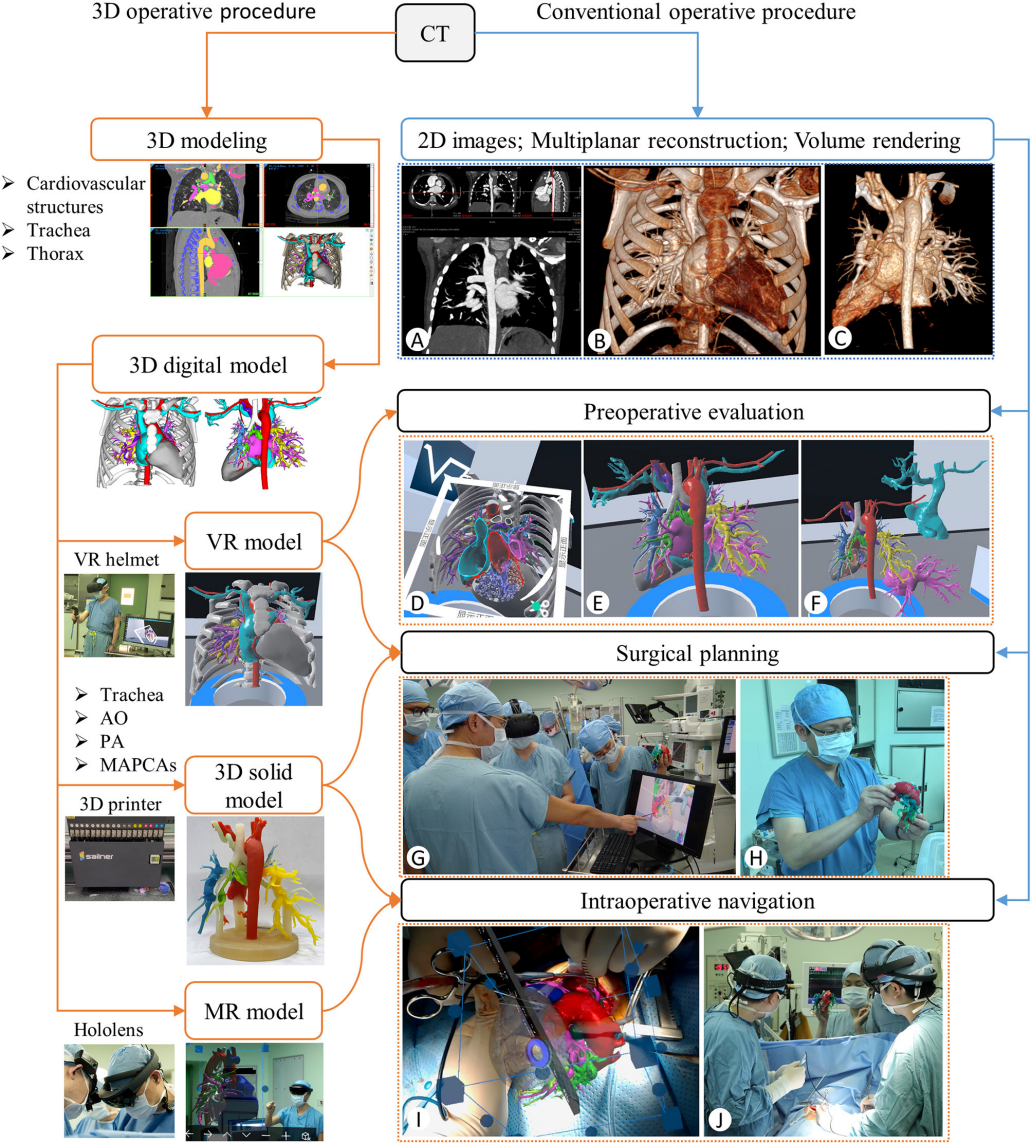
Flows of the 3D visualized (orange line) and the conventional (blue line) operative procedures. (A) CT Multiplanar Reconstruction to review the anatomy. (B,C) CT Volume rendering with and without the thorax part. (D–F) Preoperative evaluation with the section, rotation, and separation of the VR models. (G,H) Surgical planning with the VR and 3D solid models. (I,J) Determination of the MAPCAs location by coinciding the virtual and real hearts on the Hololens device (I) and anatomical review by observing the 3D solid model placed next to the operating table (J) during the operation.
